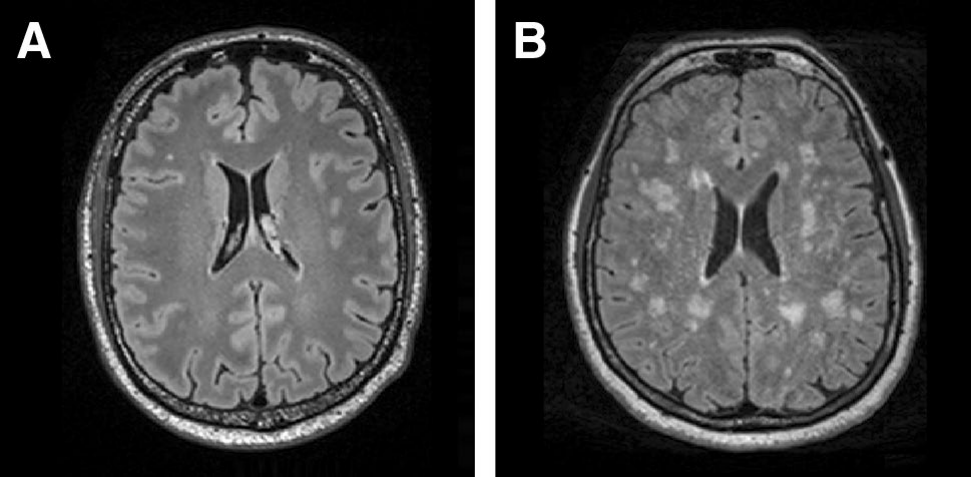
Example of different WMH volumes. Axial view of the T2-weighted fluid attenuated inversion recovery data of a subject with low WMH volume of 58 mm^3^ (A) and of a subject with high WMH volume of 31,300 mm^3^ (B).
